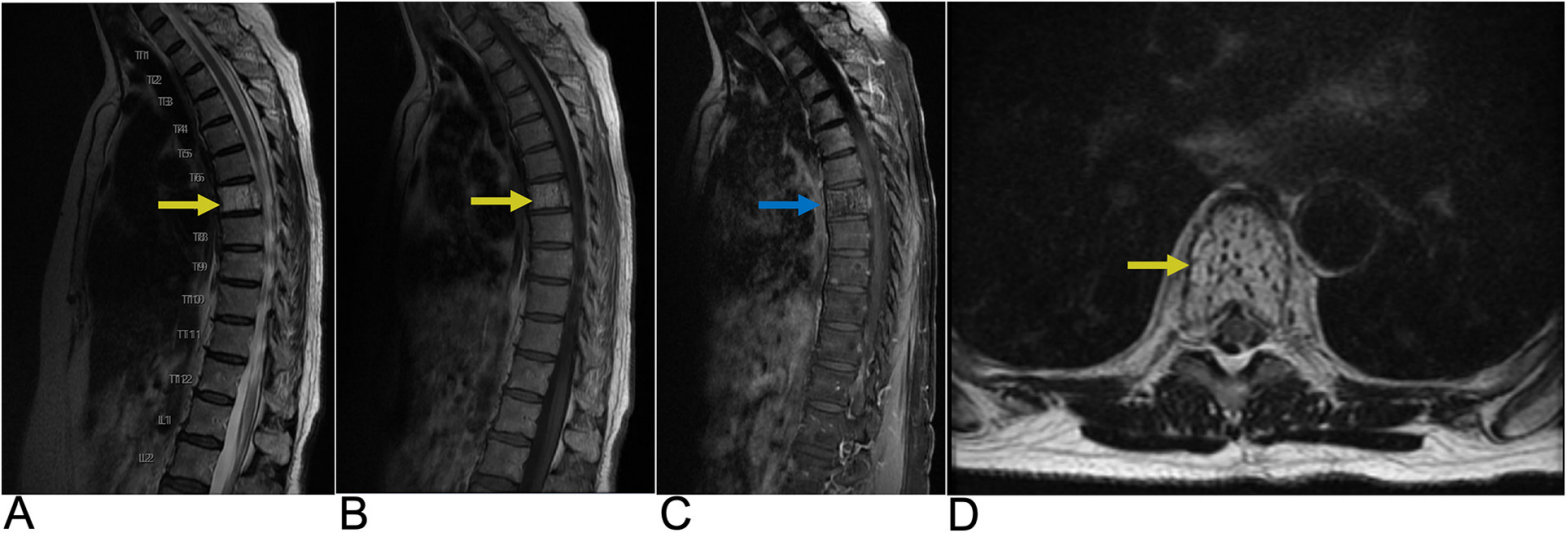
Vertebral hemangioma. Sagittal T2 (A), sagittal T1 (B), sagittal T1 FAT + C (C), and axial T2 (D) MRI of the thoracic spine demonstrating a granular “salt and pepper” T1 & T2 hyperintense (yellow arrows), heterogeneously enhancing (blue arrow) lesion representing hemangioma of the T7 vertebral body.
